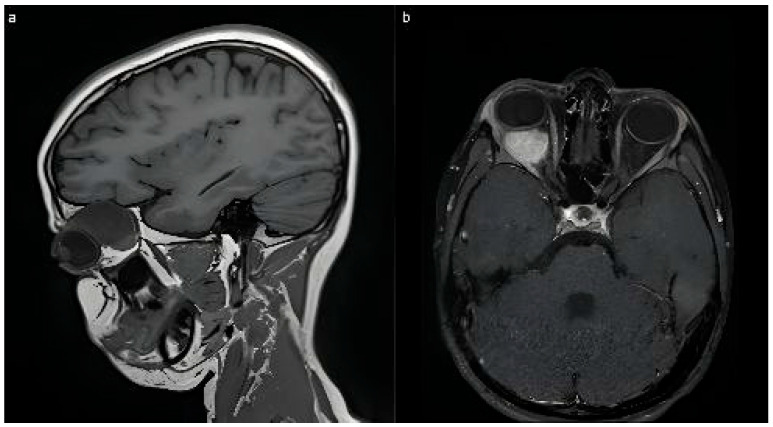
MRI image of an Intraconal tumor affecting the right eye. Note the extension of the lesion in the orbital apex and the clear margins of the tumor. (a) sagittal; (b)axial view.
